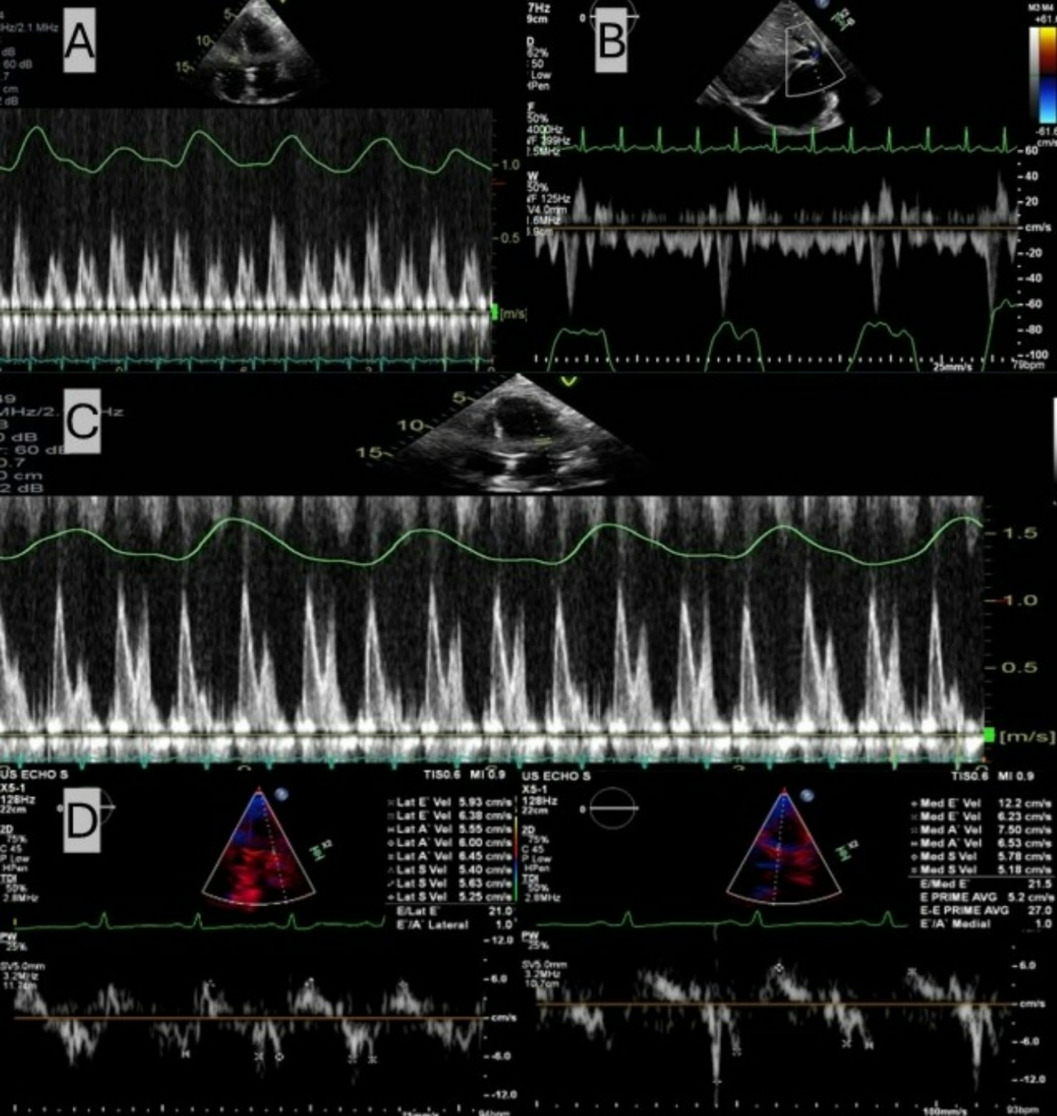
Transthoracic pulsed-wave Doppler analysis. (A) apical 4-chamber view showing respirophasic change of the tricuspid inflow velocities; (B) subcostal view showing pleural effusion and hepatic venous Doppler with expiratory diastolic flow reversal and increased forward flow; (C) apical 4-chamber view showing the mitral inflow velocities without respirophasic change; (D) tissue Doppler showing annulus reversus
